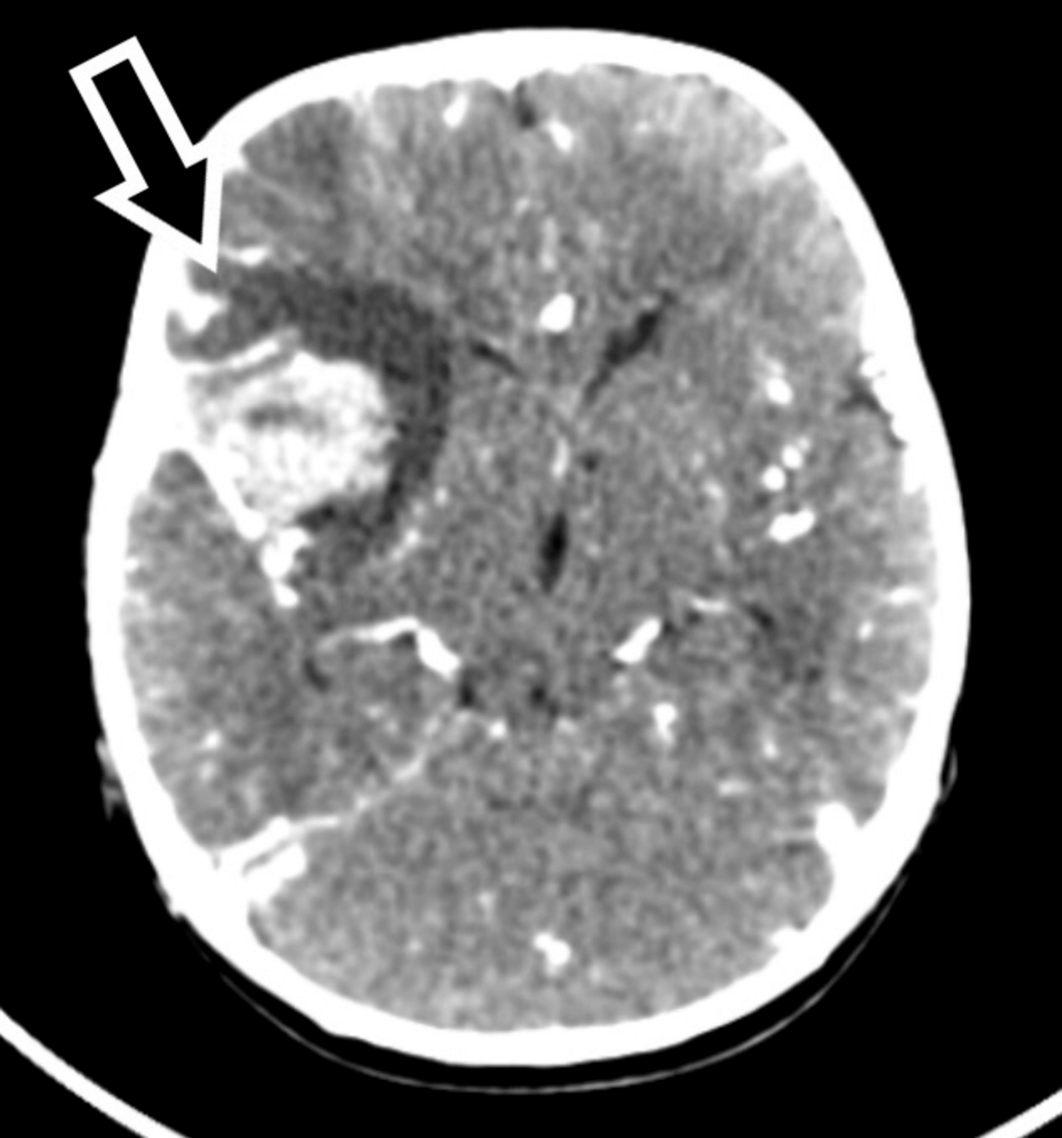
Brain computed tomography angiogram (axial section) showing intraparenchymal hemorrhage in the right frontotemporal lobe (right MCA territory) with adjacent subarachnoid component (black arrow) and edema causing a midline shift to the right side. MCA, middle cerebral artery
